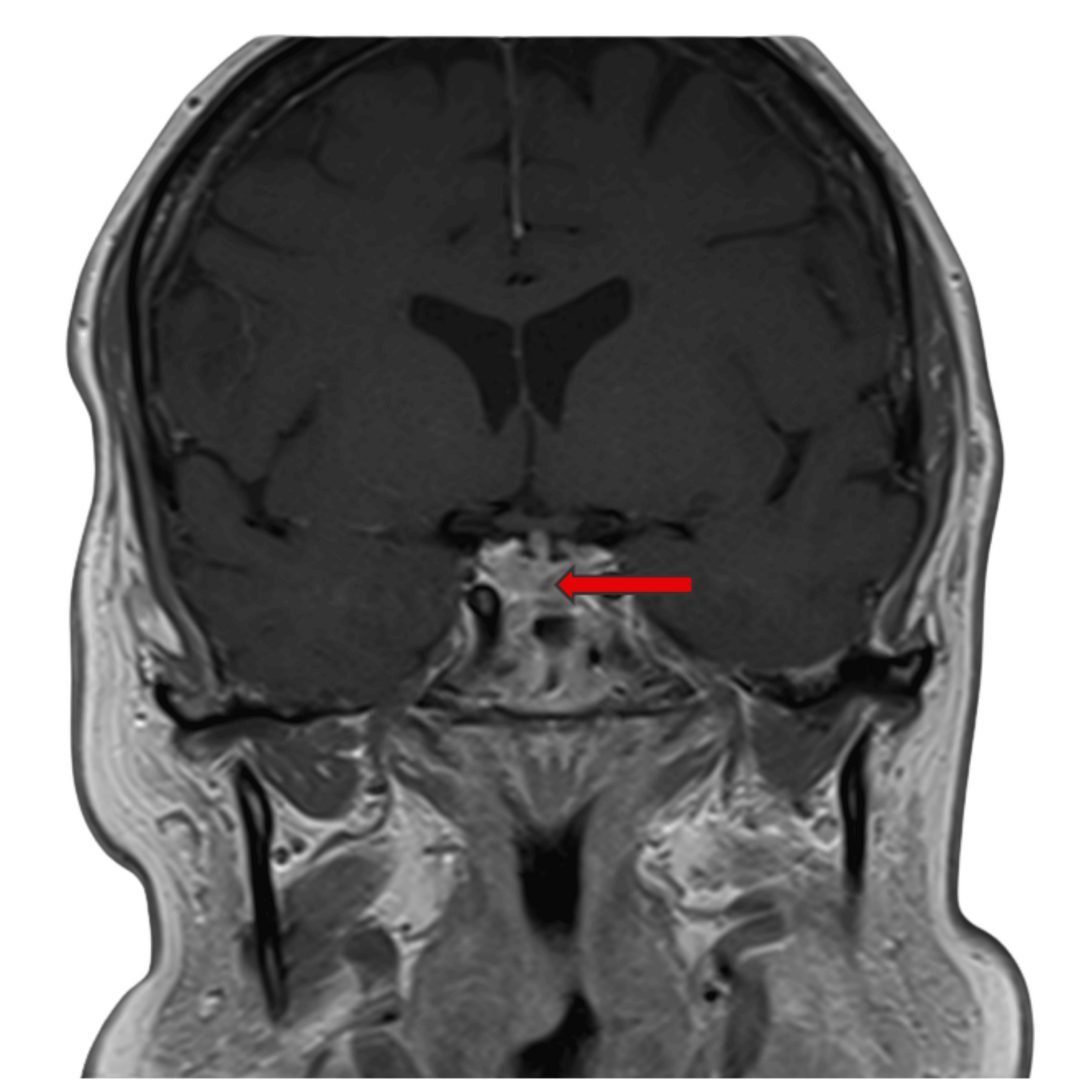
Coronal post-contrast pituitary MRI showing a 2 mm hypoenhancing microadenoma (MA) in the anterior lobe
